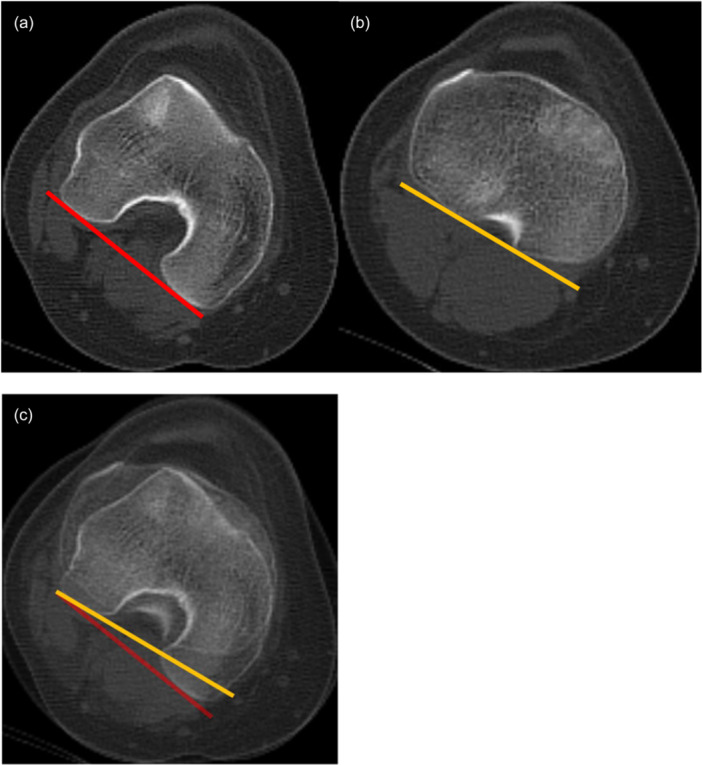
The figure shows the measurement of the TFR angle on CT, right knee, same patient as in Figure 1. (a) The posterior femoral condylar line (red) is drawn at the level of the widest anterior–posterior diameter of the condyles, in the closest slice where both posterior condylar cartilages could be appreciated. (b) The posterior tibial condylar line (yellow) is drawn at the first slice below the menisci, at the level of the posterior cruciate ligament insertion. (c) The two images were superimposed. The TFR angle was calculated as the angle between the two lines (in this example 9°; the tibia is externally rotated in relation to the femur). CT, computed tomography; TFR, tibiofemoral rotation.
